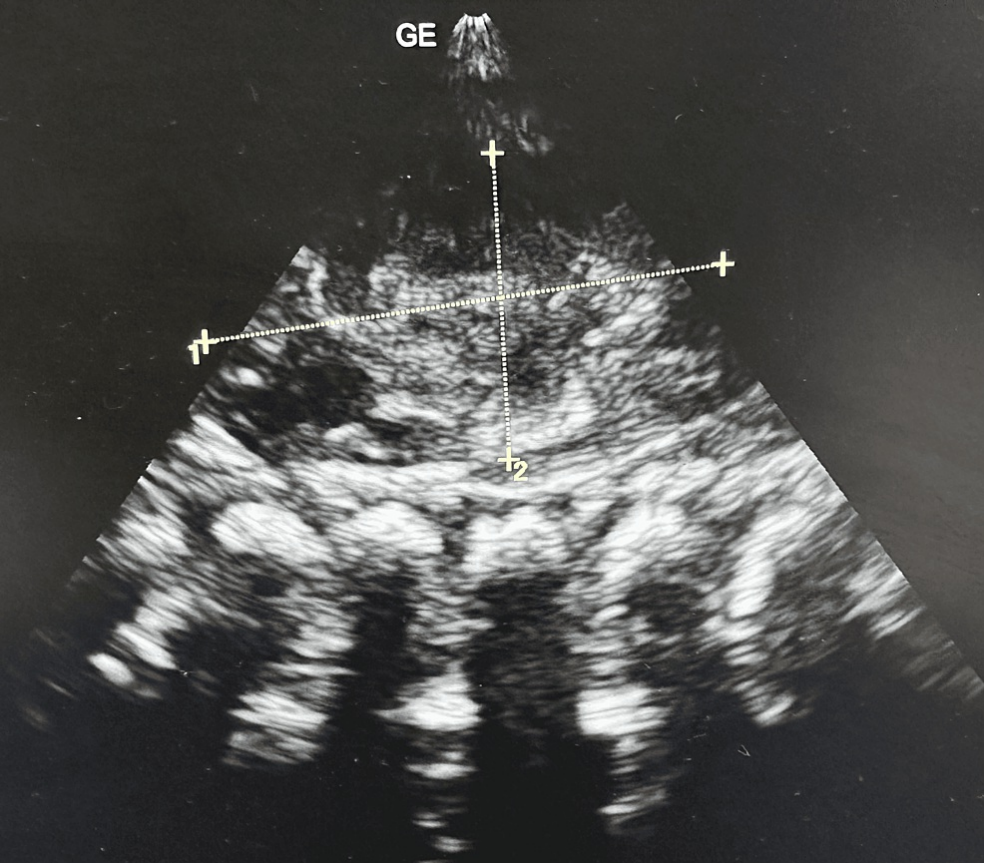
Follow-up grayscale ultrasound image showing normal anechoic renal medulla.
